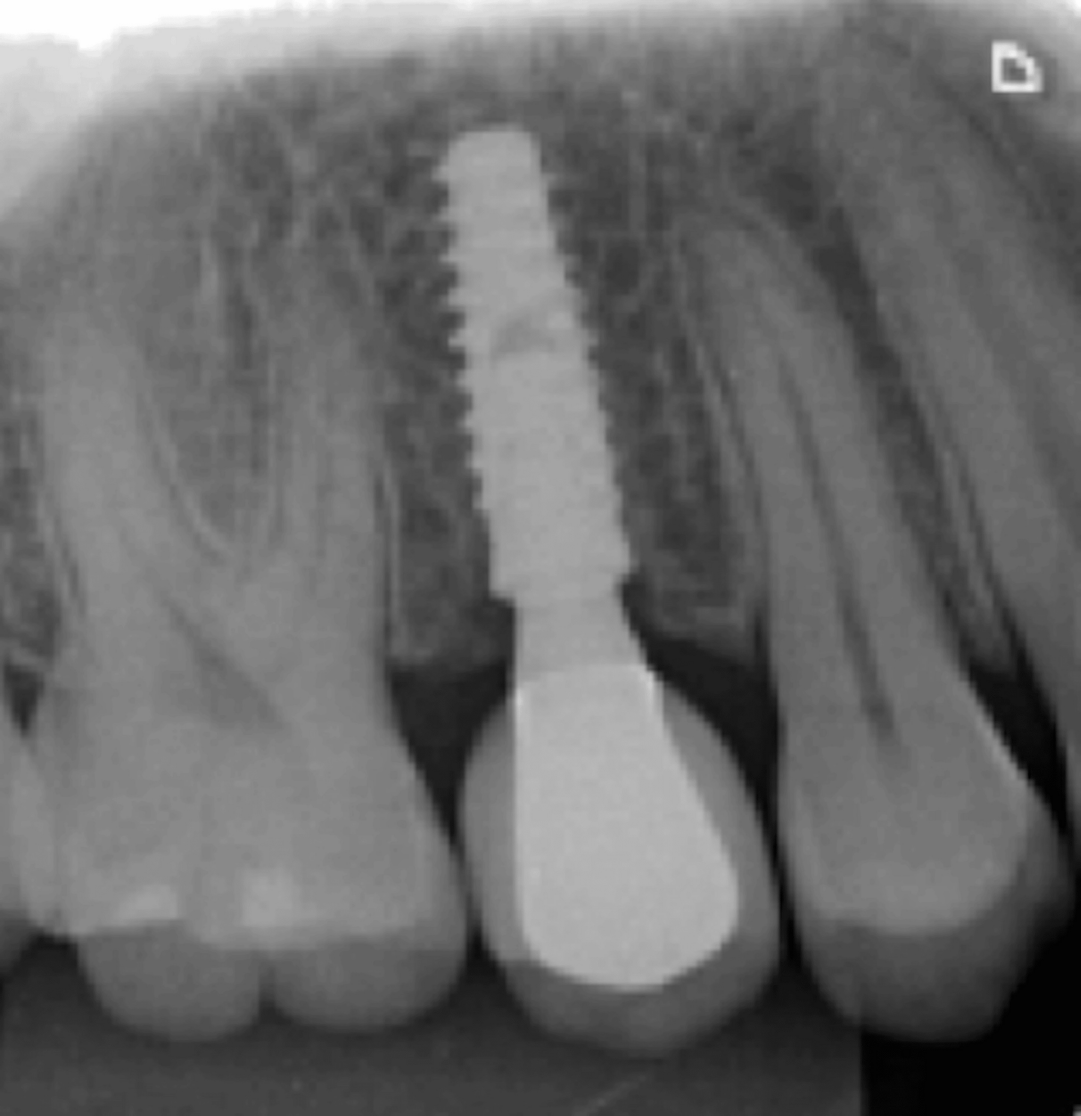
Control radiograph of implant 15 with the ceramic screw-retained crown
